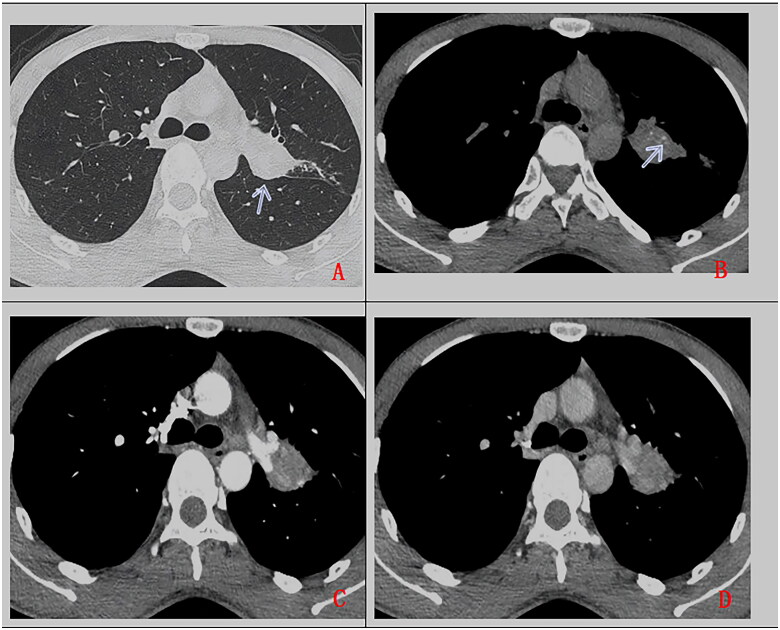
A 27-year-old man, with cough for more than 2 months. Chest CT scan without contrast (A. lung window, B. mediastinum window) and with contrast (C. artery phase, D. delayed phase). Soft tissue mass in left upper hilum (as indicated by arrow), with lobulated margin, heterogeneous density, punctuate calcification (as indicated by arrow), uneven enhancement, accompanied by adjacent bronchus obstruction. Pathologically confirmed PMEC after surgical operation.
